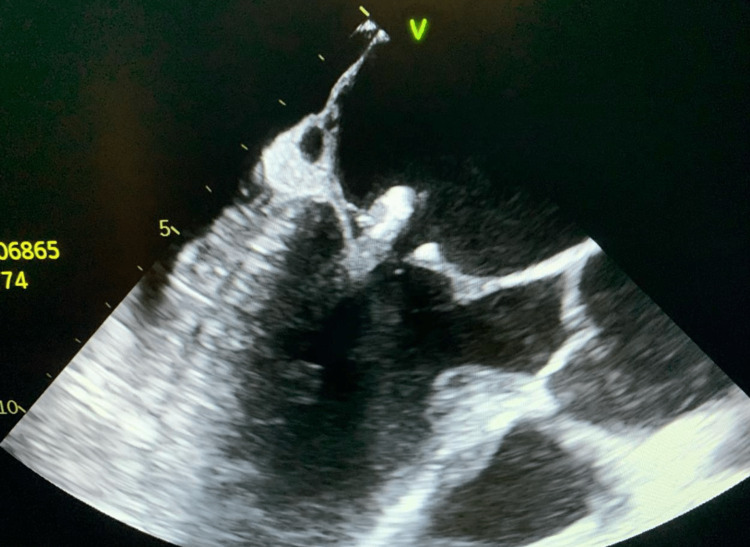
Transesophageal Echocardiogram Performed Demonstrating a Posterior Mitral Valve Leaflet Vegetation
